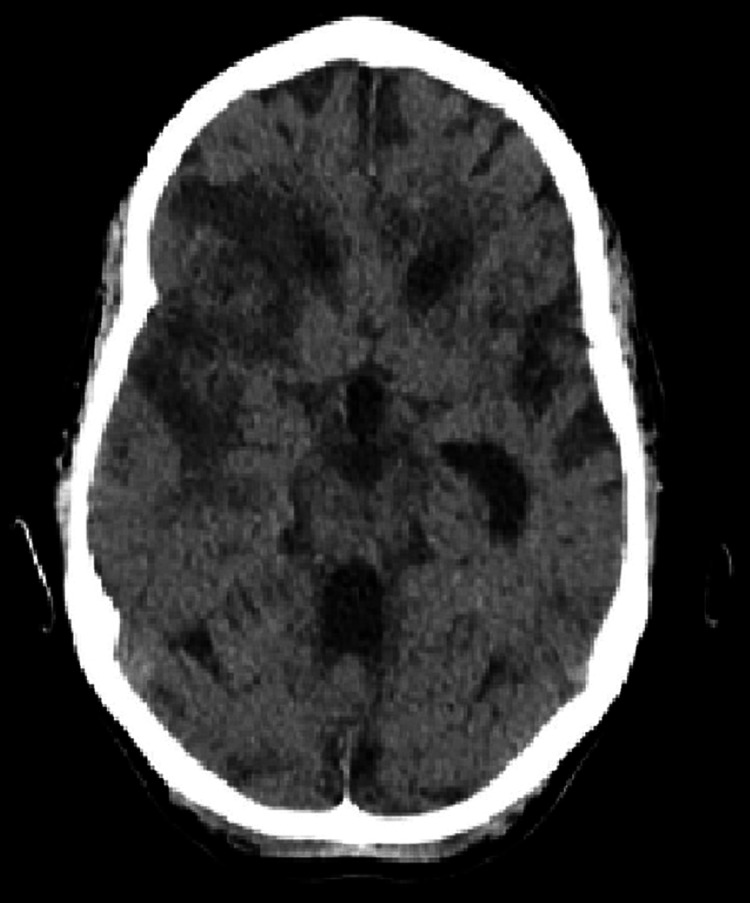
Axial CT brain on day four of admission, demonstrating a moderate mass effect with effacement of the posterior right lateral ventricle with associated midline shift.
